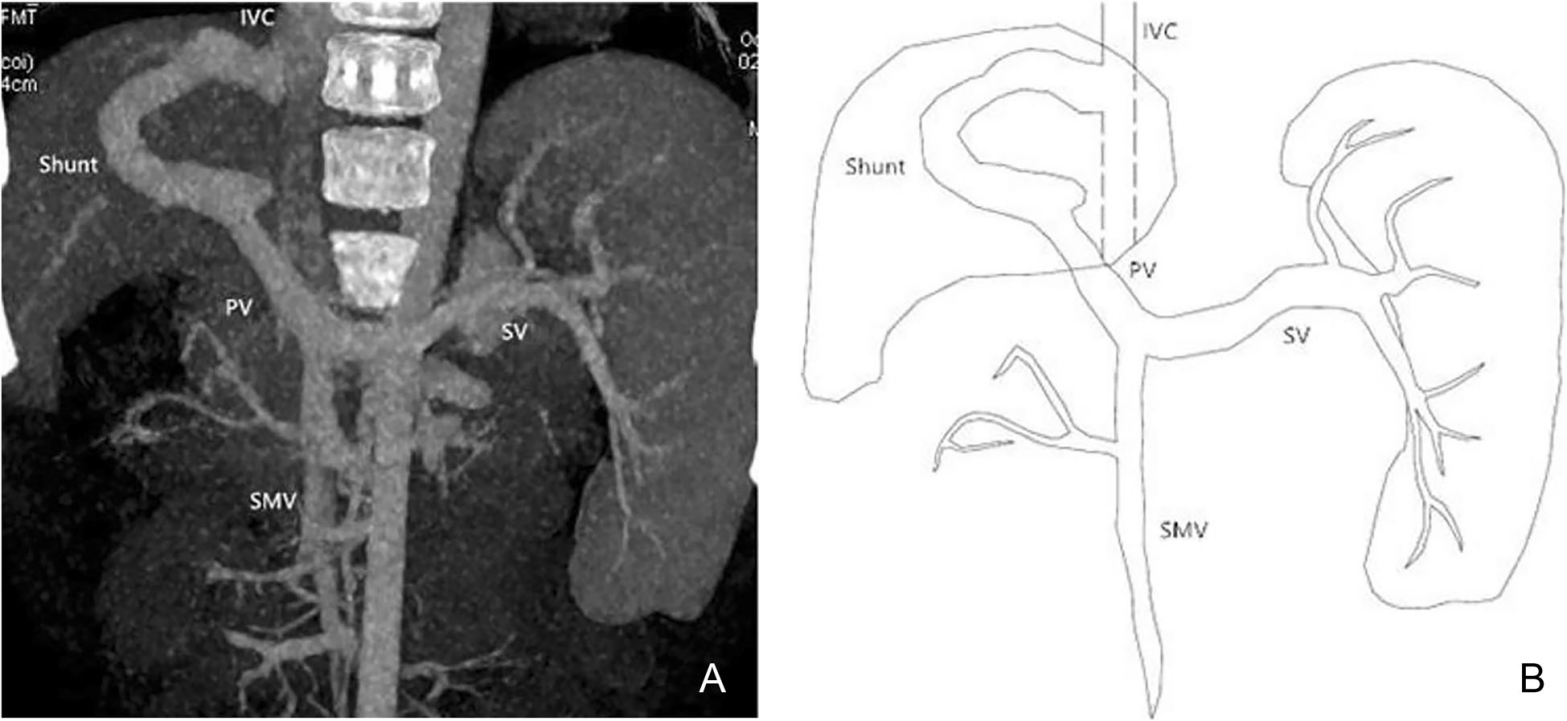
Male, 7 years old, Abernethy II. Abdominal CT angiography maximum intensity projection (MIP) image (portal phase) shows the shunt of extrahepatic portosystemic between portal vein and inferior vena cava
